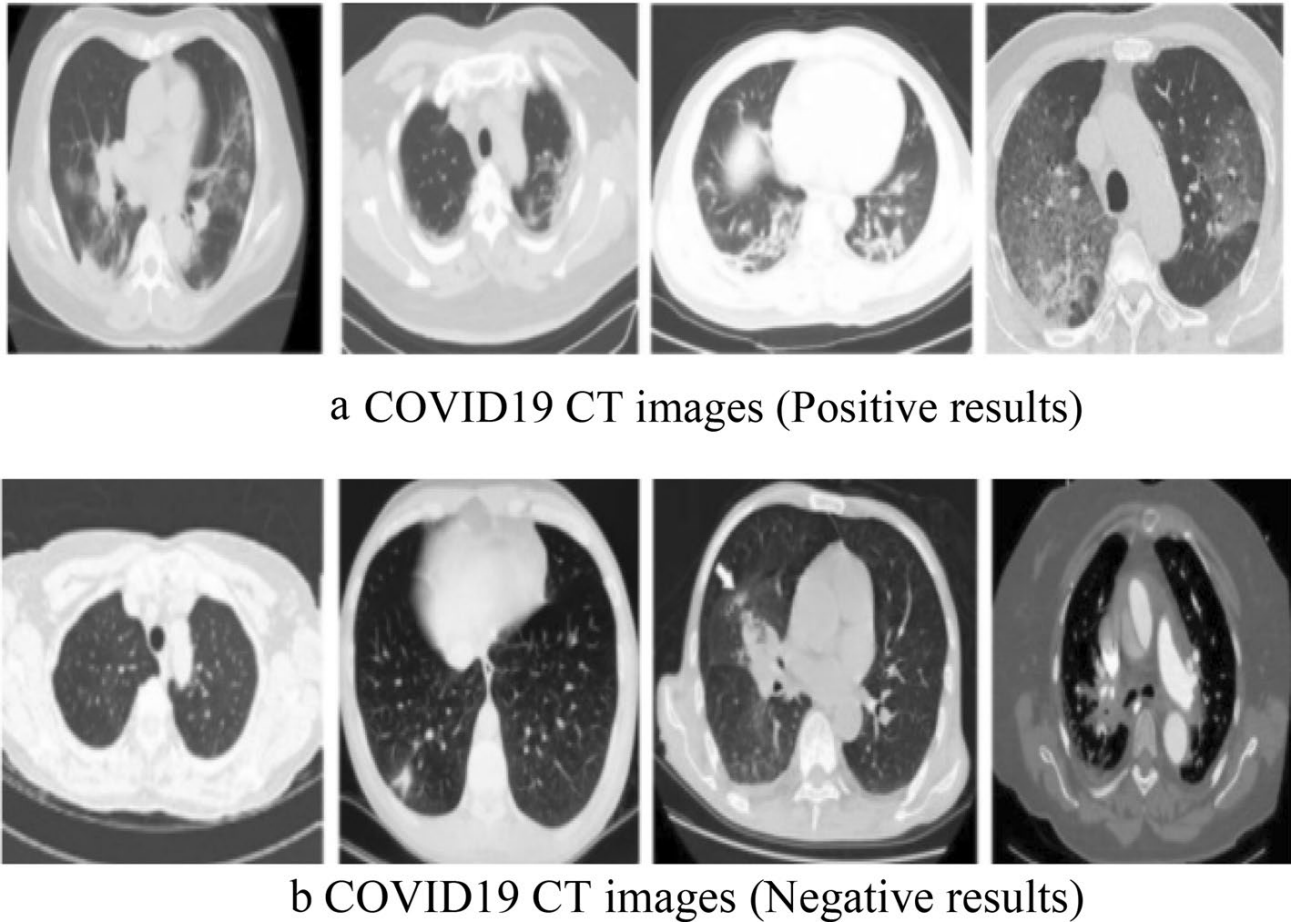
Samples of COVID19 enhanced CT images.
